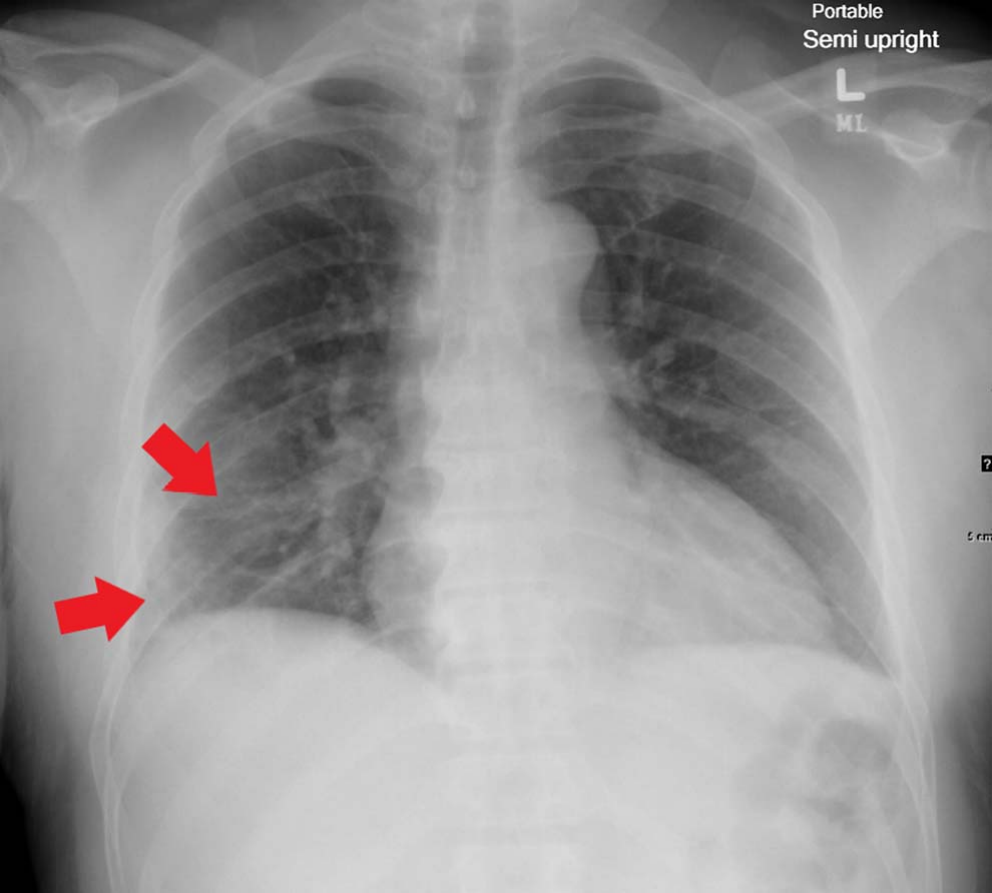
Portable semi-upright anteroposterior radiograph of a 56-year-old male shows unilateral thin bandlike (arrows) consolidative opacity in the periphery of the right mid and lower lung zones admixed with patchy airspace opacity. This pattern was considered atypical, but more suspicious for coronavirus disease 2019.
